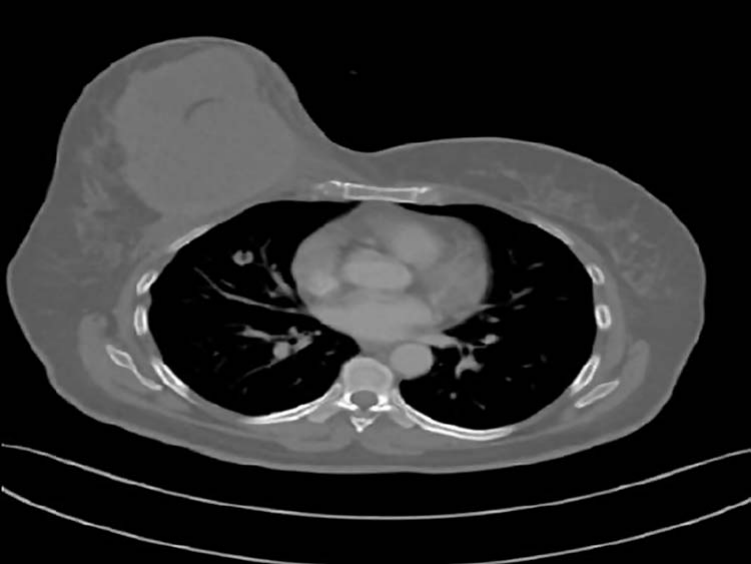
Chest CT shows a high-density mass in the right breast infiltrating the chest wall, accompanied with a metastatic lesion in the right lung.
